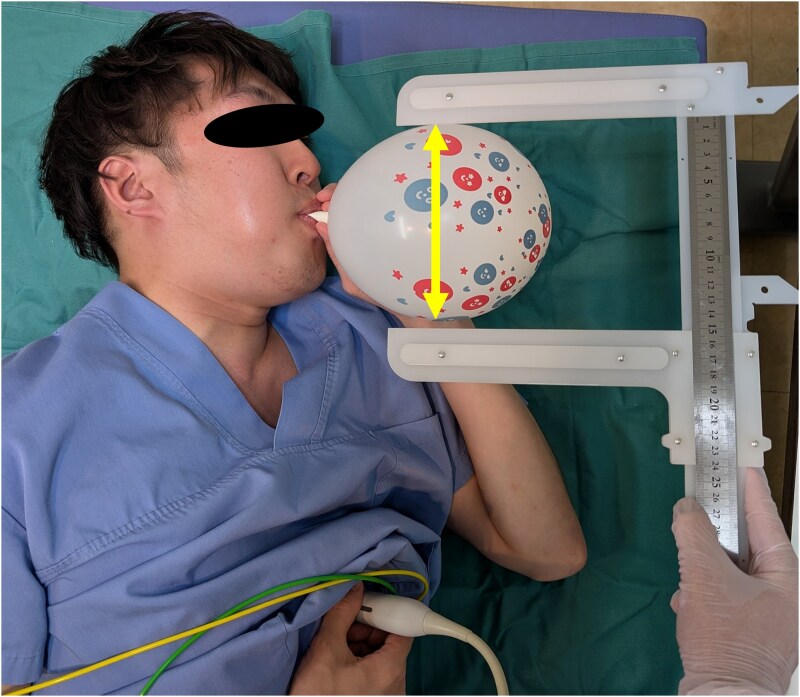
Participants performing PBIM. TTE was performed in the left lateral decubitus position while the participant inflated the party balloon according to the established protocols. The arrow indicates the diameter of the balloon measured using the vernier calliper. TTE, transthoracic echocardiography.
